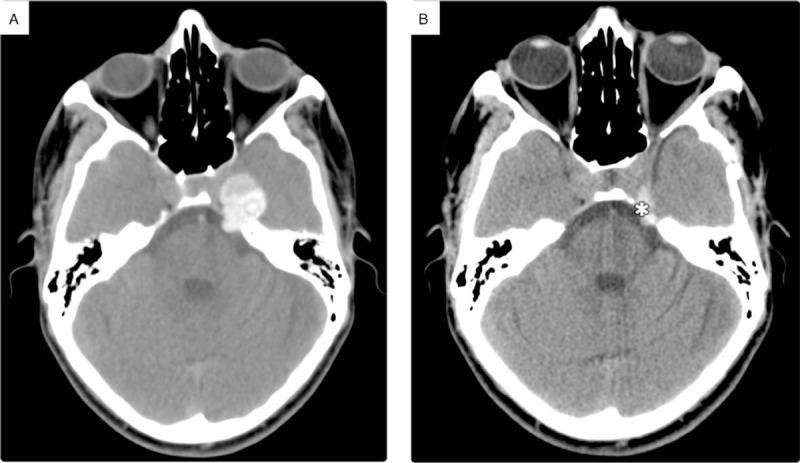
Unenhanced axial computed tomography scans of the brain. (A) Heterogeneously hyperdense mass lesion occupying the left Meckel cave inducing mass effect along the ipsilateral lateral cavernous sinus and extending to a cisternal portion of the trigeminal nerve. (B) Follow-up post subtotal resection through left temporal craniotomy with the small residual lesion (asterisk).
